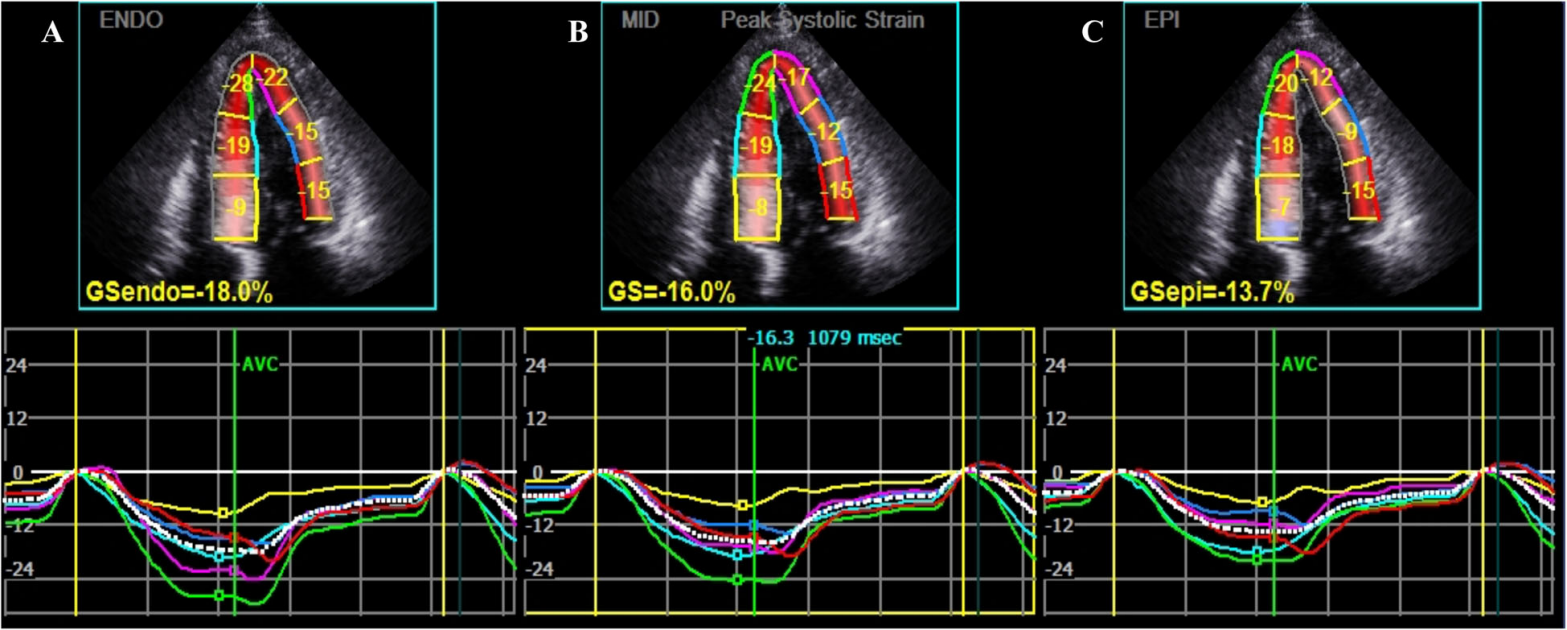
Representative images of two-dimensional (2-D) left ventricular (LV) global layer-specific strain (LSS) analysis in a patient with hypertrophic cardiomyopathy (HCM) from apical 4-chamber views. The 2-D LV global LSS was also measured from apical 2- and 3-chamber views. The analyses were performed using Echo PAC version 201. The quantitative strain measurements of the endocardial (a), middle (b) and epicardial myocardial layers (c) were automatically calculated after the region of interest was manually traced and adjusted
